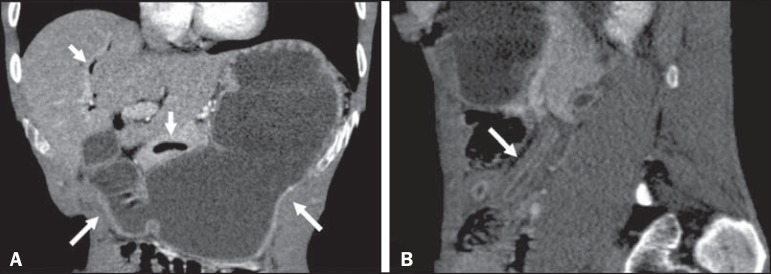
Intravenous contrast-enhanced CT scan, in the coronal and sagittal planes
